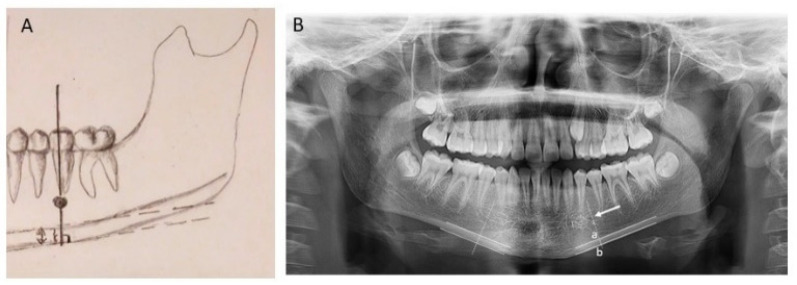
Mandibular cortical width (MCW) measurement: (A) A graphical representation of a MCW measurement. (B) A panoramic radiograph showing the measurement of mandibular cortical width. A line parallel to the long axis of the mandible and tangential to the inferior border of the mandible was drawn. A line perpendicular to this tangent and intersecting the mental foramen (dotted line) was constructed, along which the mandibular cortical width was measured. The distance between the two parallel solid lines a–b is the cortical width.
